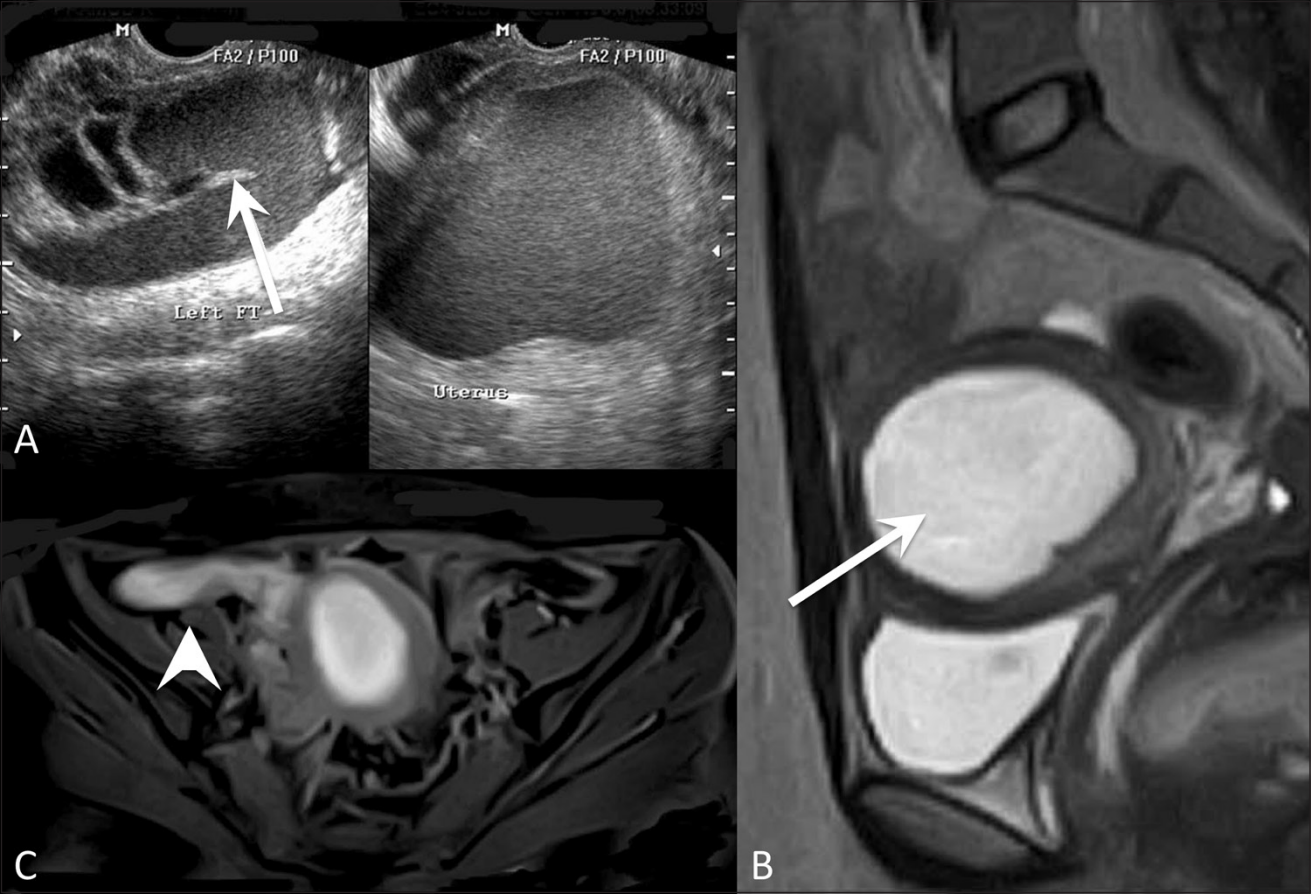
Hematometra with hematosalpinx. Transvaginal USG (A), sagittal T2W (B) and axial fat-suppressed T1W (C) MRI images show hematometra (arrow) with hematosalpinx (arrowhead) on the right side. Note incomplete septae in tube (white arrow in A)
